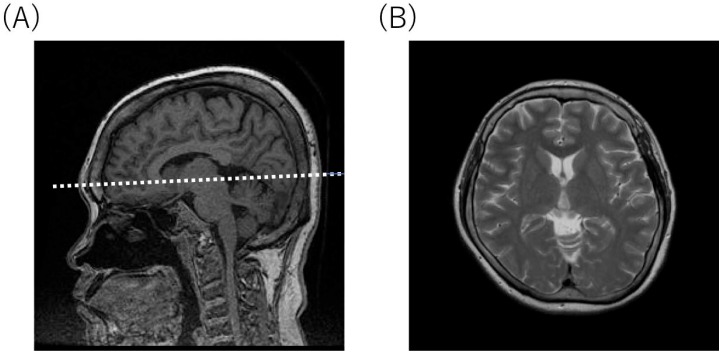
Brain MRI at 34 years of age (22 years post-HSCT). (A) Sagittal T1-weighted image. (B) Axial T2-weighted image. The white dotted line indicates the level of axial T2-weighted image shown in (B).
